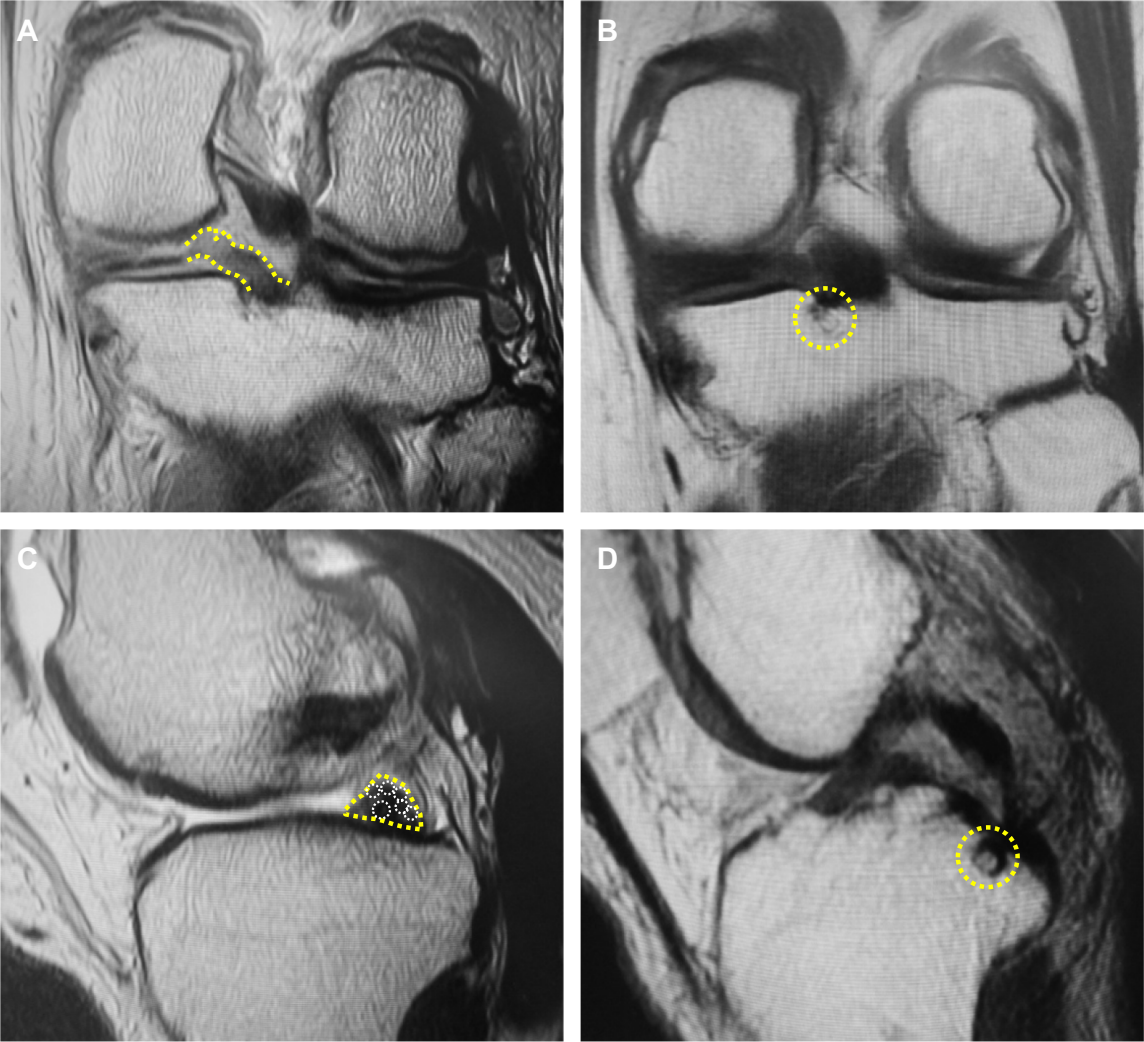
Characteristic MRI finding in partial tear/damage of the MM posterior root. A A root irregularity (dotted area, coronal view). B A bone marrow spot (subcortical cyst) under the posterior root attachment (dotted circle, coronal view). C An ocarina sign (dotted area, sagittal view nearby the MM posterior root). D A bone marrow spot (dotted circle, sagittal view)
